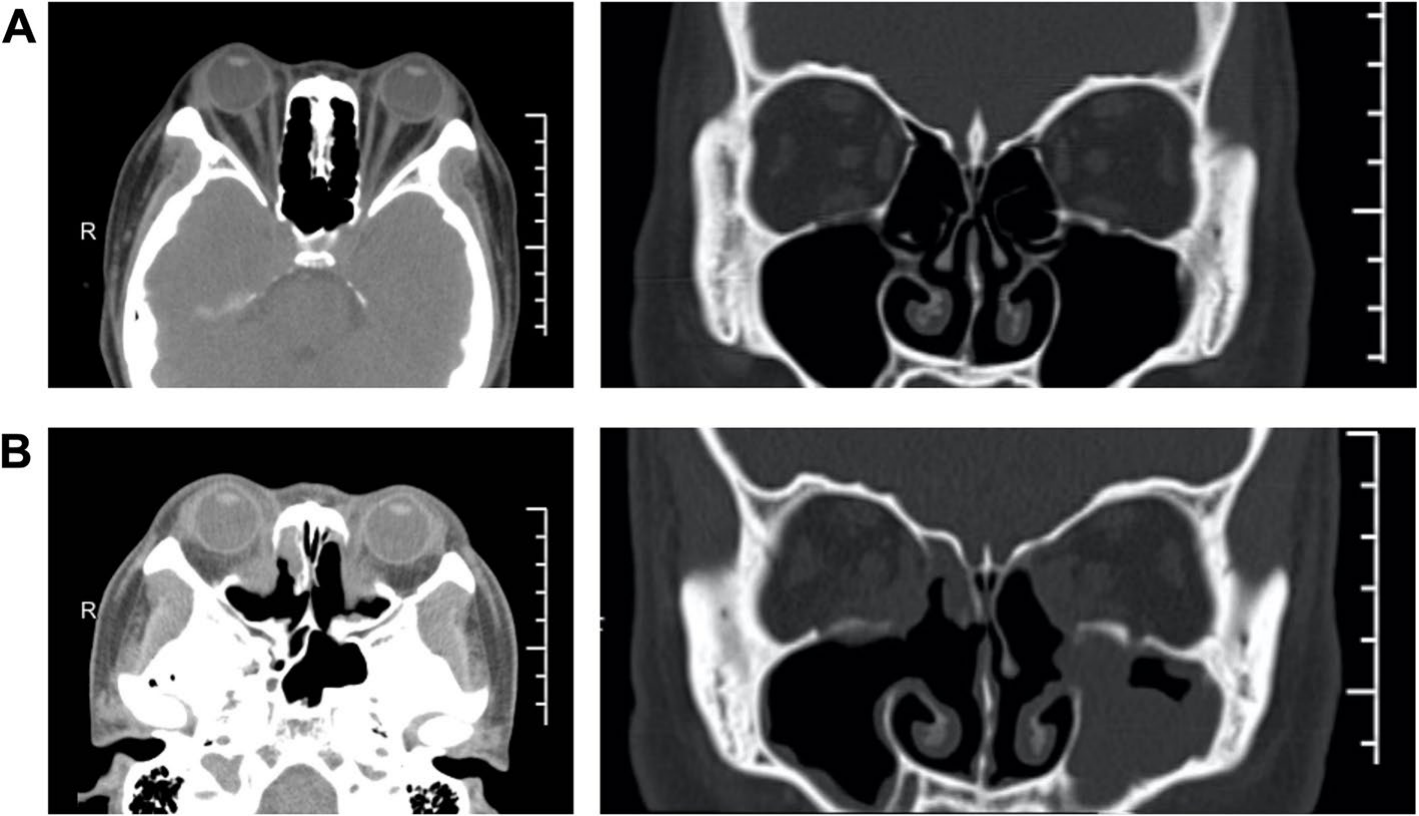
CT imaging before and after surgery. A Horizontal and coronal CT images before surgery. CT scans revealed binocular protrusion, increased orbital adipose tissue, and crowding of the orbital apex. B Horizontal and coronal CT images after surgery. A postoperative orbital CT revealed that the exophthalmos had significantly receded, most of the bone in the medial orbital wall's posterior part had vanished, the middle and posterior parts of the orbital cavity had enlarged, the fat herniation was uniform, and the extraocular muscle was not incarcerated
